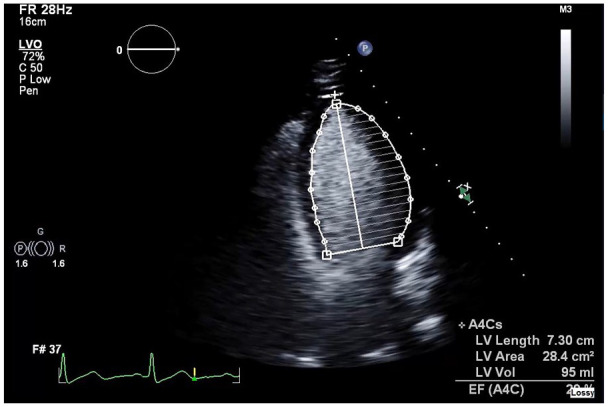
Echocardiogram on admission revealing dilation of the left ventricle.
